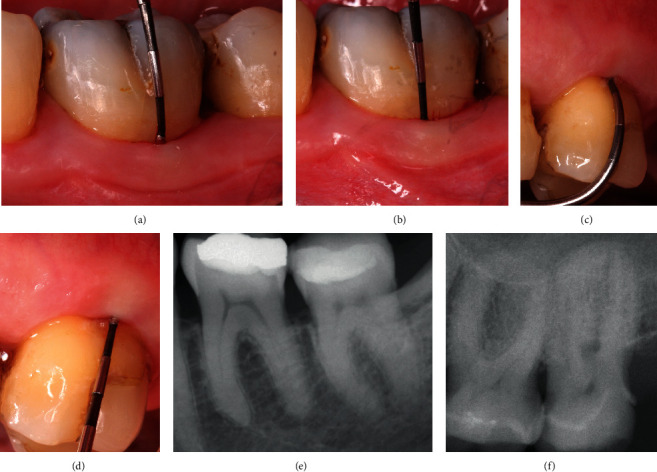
(a) Months' observation at tooth 36 reveals a 2 mm buccal HPD indicating the relevant improvement in the furcation area. (b) 12-month buccal VPD at 36 equals 3 mm depth, displaying a valuable improvement in vertical dimension. (c) 18-month observation displays a 3 mm of horizontal penetration depth (HPD) at tooth 26, indicating conversion from FI grade 2 to grade 1. (d) 18-month observation displays a 3 mm of vertical penetration depth (VPD) at tooth 26n from the buccal, indicating clinically relevant improvement in this dimension. (e) Periapical radiograph of tooth 36 after 12 months confirms clinical assessments and corroborates the improvement in the furcation area. (f) Periapical radiograph of tooth 26 after 30 months corroborates clinical measurements and confirms the success of the treatment.
